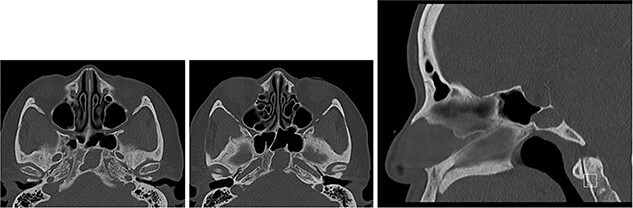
CT of the base of skull (axial images ×2 and sagittal image) showing sphenoid mucocele and paper-thin posterior cortex.
